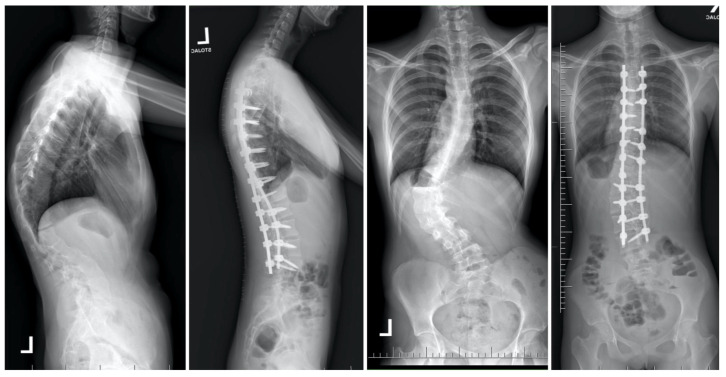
Radiographs taken before and after surgery at the last follow-up appointment depicting a 14-year-old female with severe adolescent idiopathic scoliosis who underwent intraoperative halo-femoral traction, followed by multi-level Ponte osteotomy and posterior spinal fusion in a single-stage surgical procedure.
